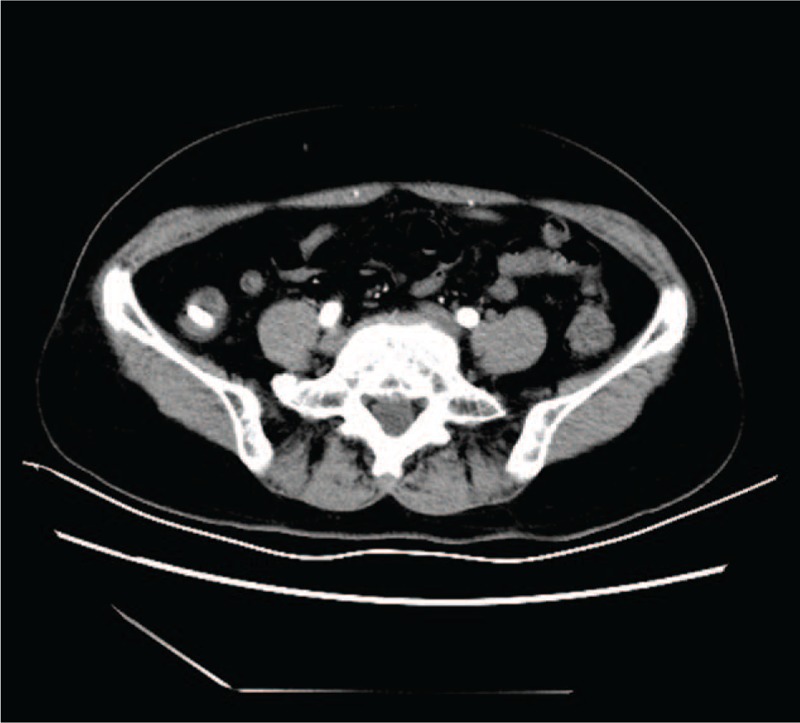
Computed tomography (CT) scan showing a high-density nodular shadow in the cecal cavity, approximately 0.8 cm×1.5 cm in size.
